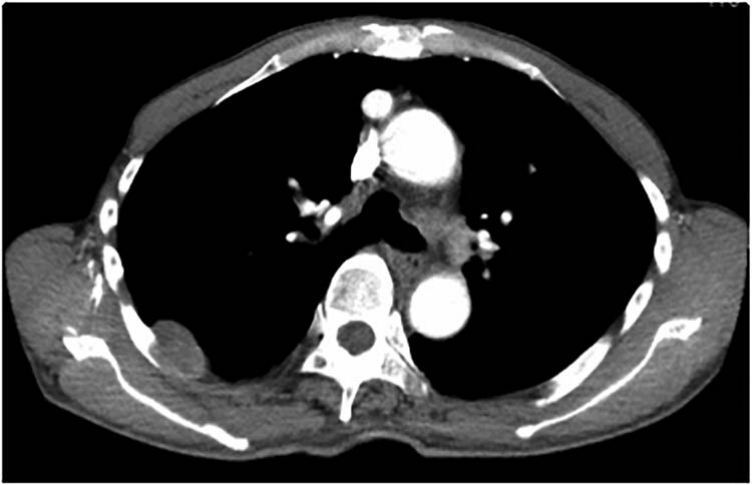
Contrast-enhanced chest CT showing a smooth surface of 3 cm.
